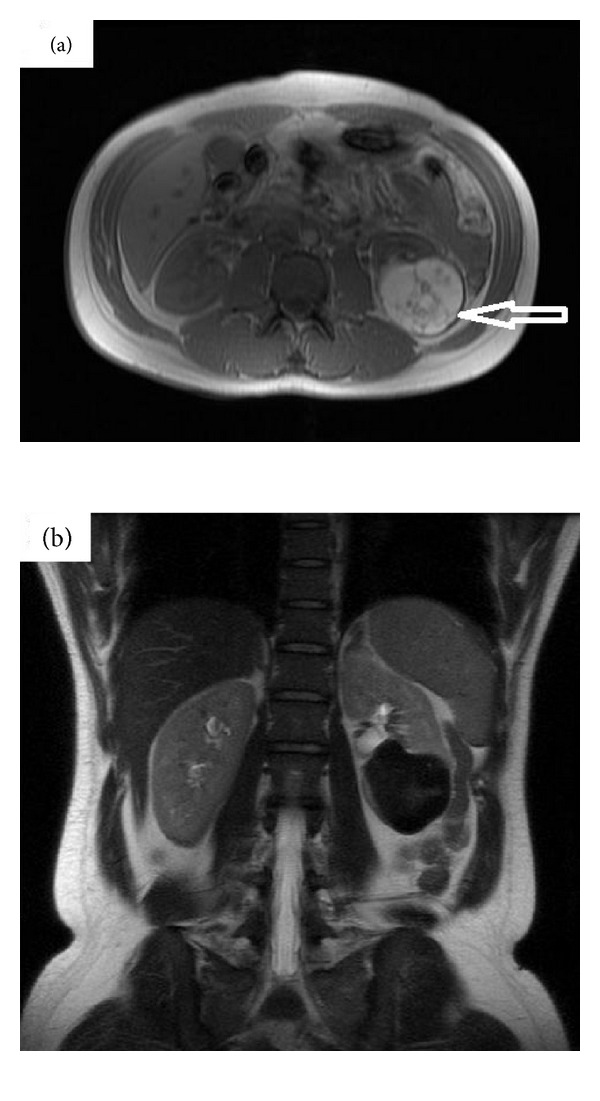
(a) T1-weighted MRI in axial section showing left renal mass with bright signal (see arrow). (b) Left renal lesion is black on this T2-weighted coronal section MRI. No haemorrhagic component was seen.
